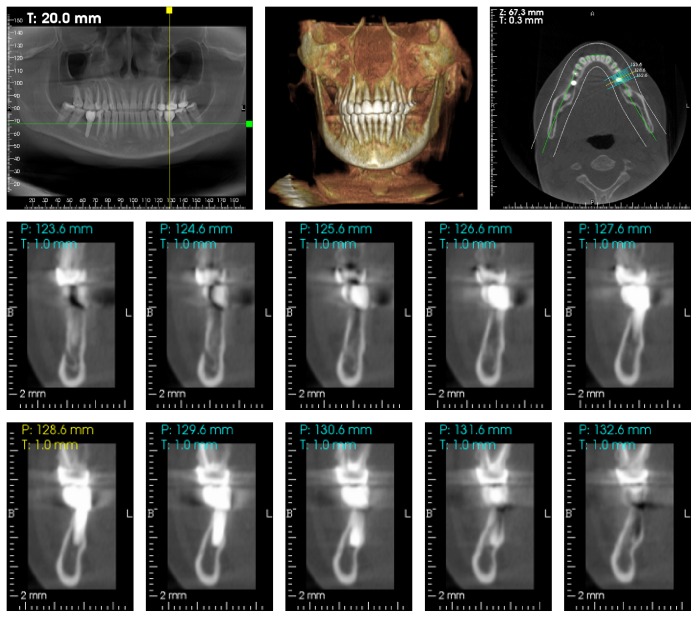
Cbct. 2 years after loading.
